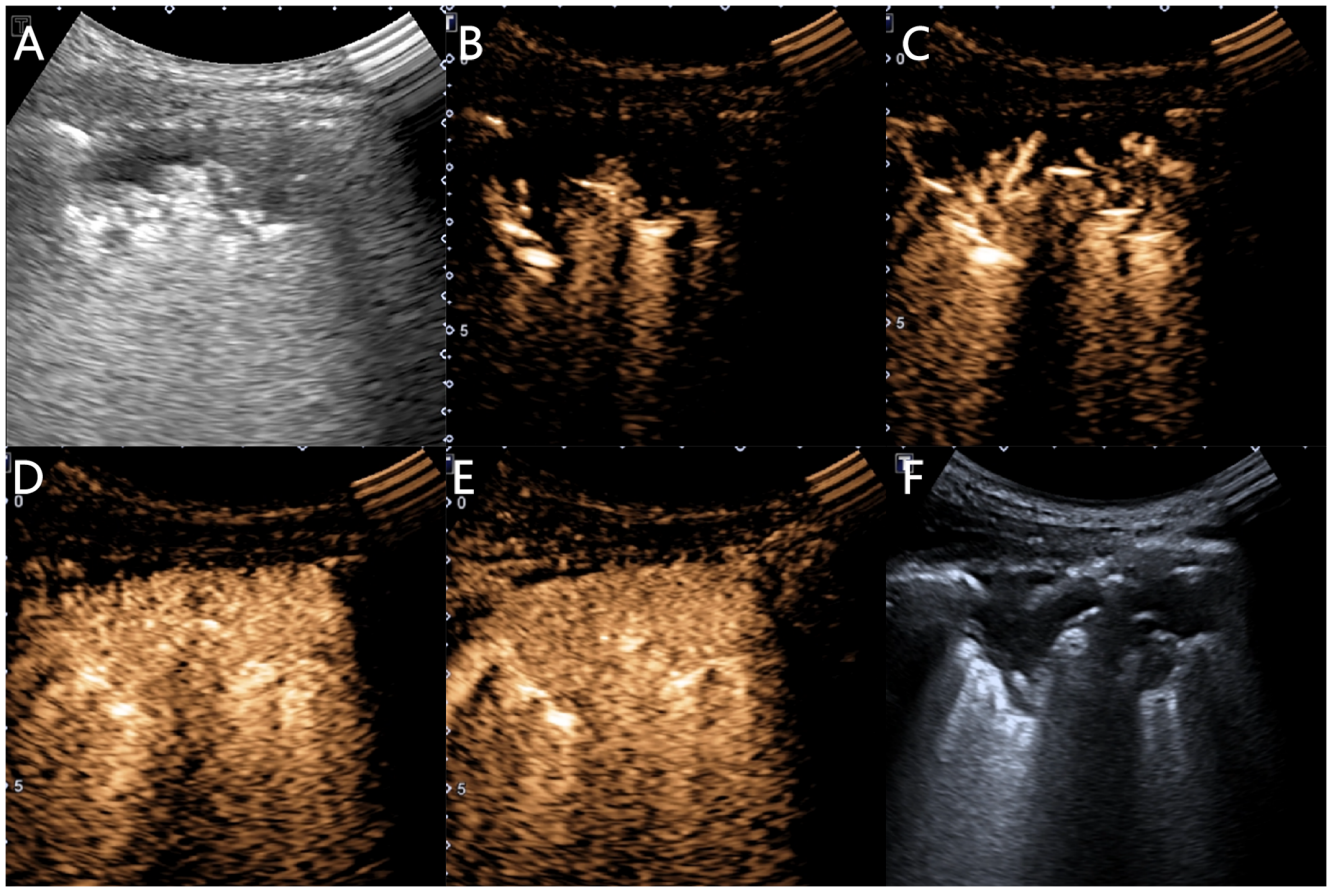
Ultrasound images of an 81-year-old female patient with pneumonia. (A) A hypoechoic solid lesion with an irregular shape and blurred edges, measuring 5.4 × 1.9 cm, can be seen in the right lung. (B) At 4.1 s, the inflated lung tissue begins to enhance. (C) At 5.0 s, the lesion begins to enhance unidirectionally in a branching radicular pattern. (D) At 12.6 s, the chest wall begins to enhance. (E) The lesion shows uniform isointense enhancement, with no necrosis inside. (F) A 16G needle is used for biopsy of the lesion.
